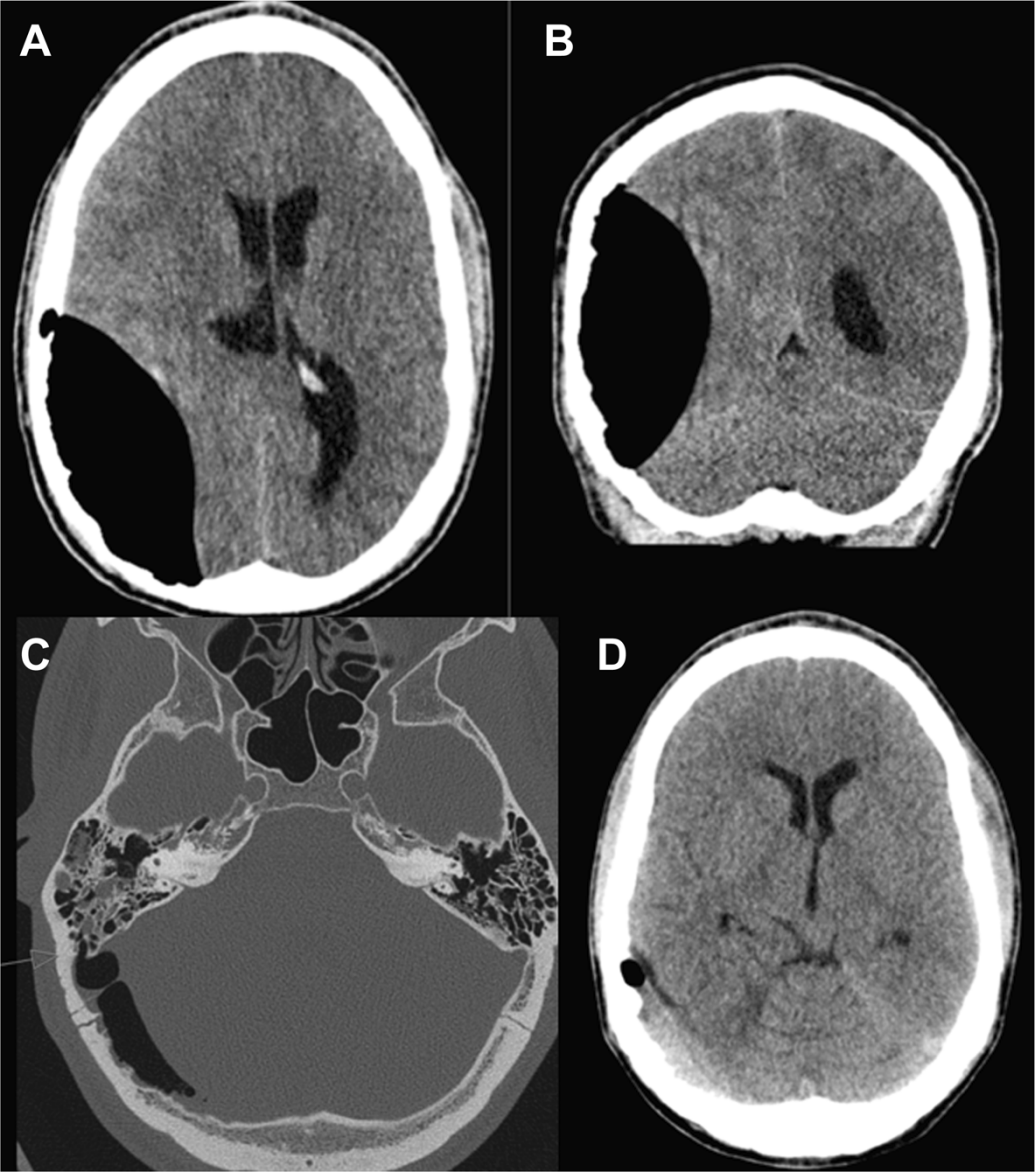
Imaging with computed tomography of the head revealed a 10cm×4cm expansive epidural lesion in the right temporoparietal region causing significant anteromedial compression of the right lateral ventricle and a midline shift of 7mm (a and b). A communication between the right mastoid sinus and the epidural space caused by a fracture was suspected (c). Postoperative control computed tomography the first postoperative day showed a small epidural bleed in the compartment that previously was filled with air, but the majority of the epidural air was removed and the midline was normalized (d)
